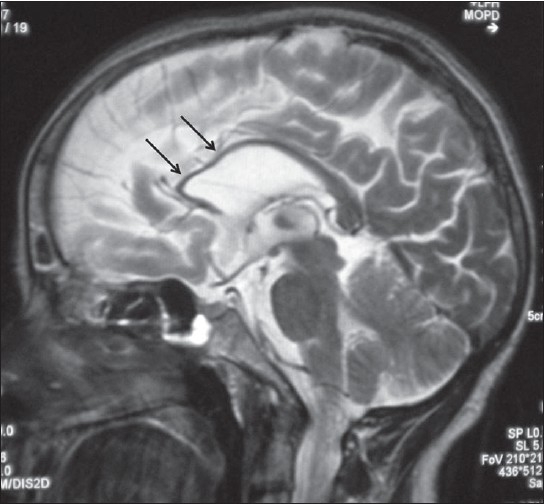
Mid-sagittal T2-weighted MR image showing thin corpus callosum mainly involving rostrum and genu (arrows) with frontoparietal lobe atrophy
